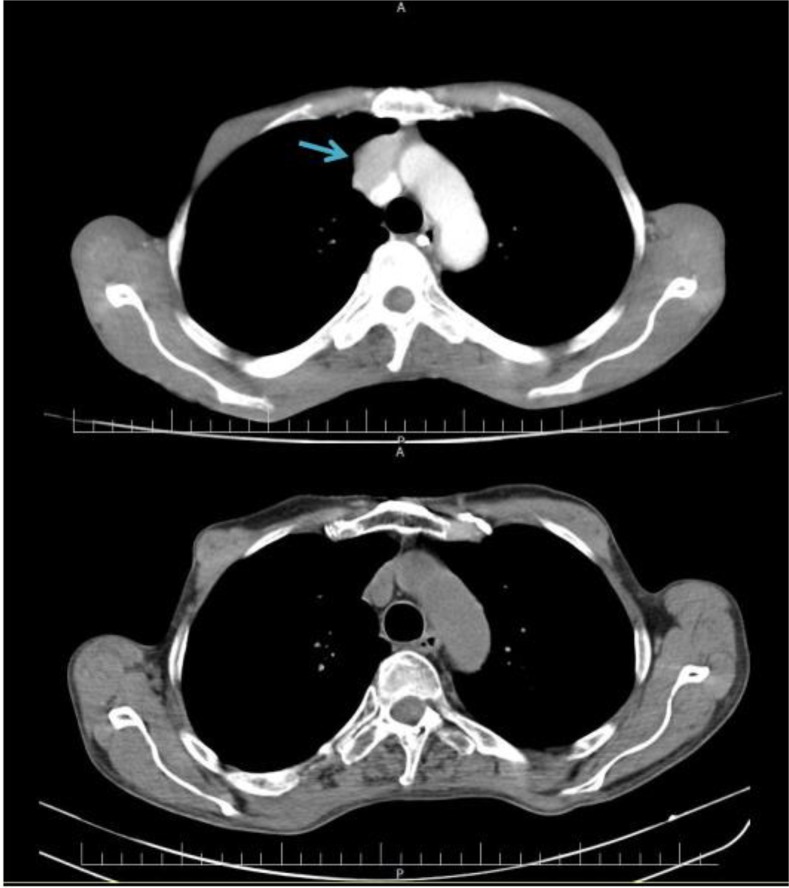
Preoperative (up) and post operative (down) spiral chest CT scan with contrast of a 60-year-old man with thymoma
